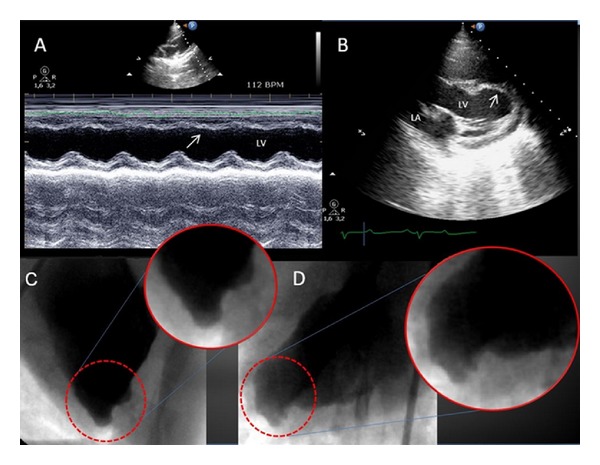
(A) Echocardiography at 1 month: M-mode image of left ventricle (LV); the arrow points at the anterior interventricular septum, which appears severely hypokinetic/akinetic, in comparison with the posterior wall. (B) Two-dimensional parasternal long axis; the LV apex appears remodeled and aneurysmatic (arrow), and wall thickness is markedly reduced in comparison with the basal segment. LA: left atrium. Follow-up ventriculography (1 month), with magnified views, showing a remodeled and aneurysmatic LV apex, in the right oblique (C) and anteroposterior (D) views.
